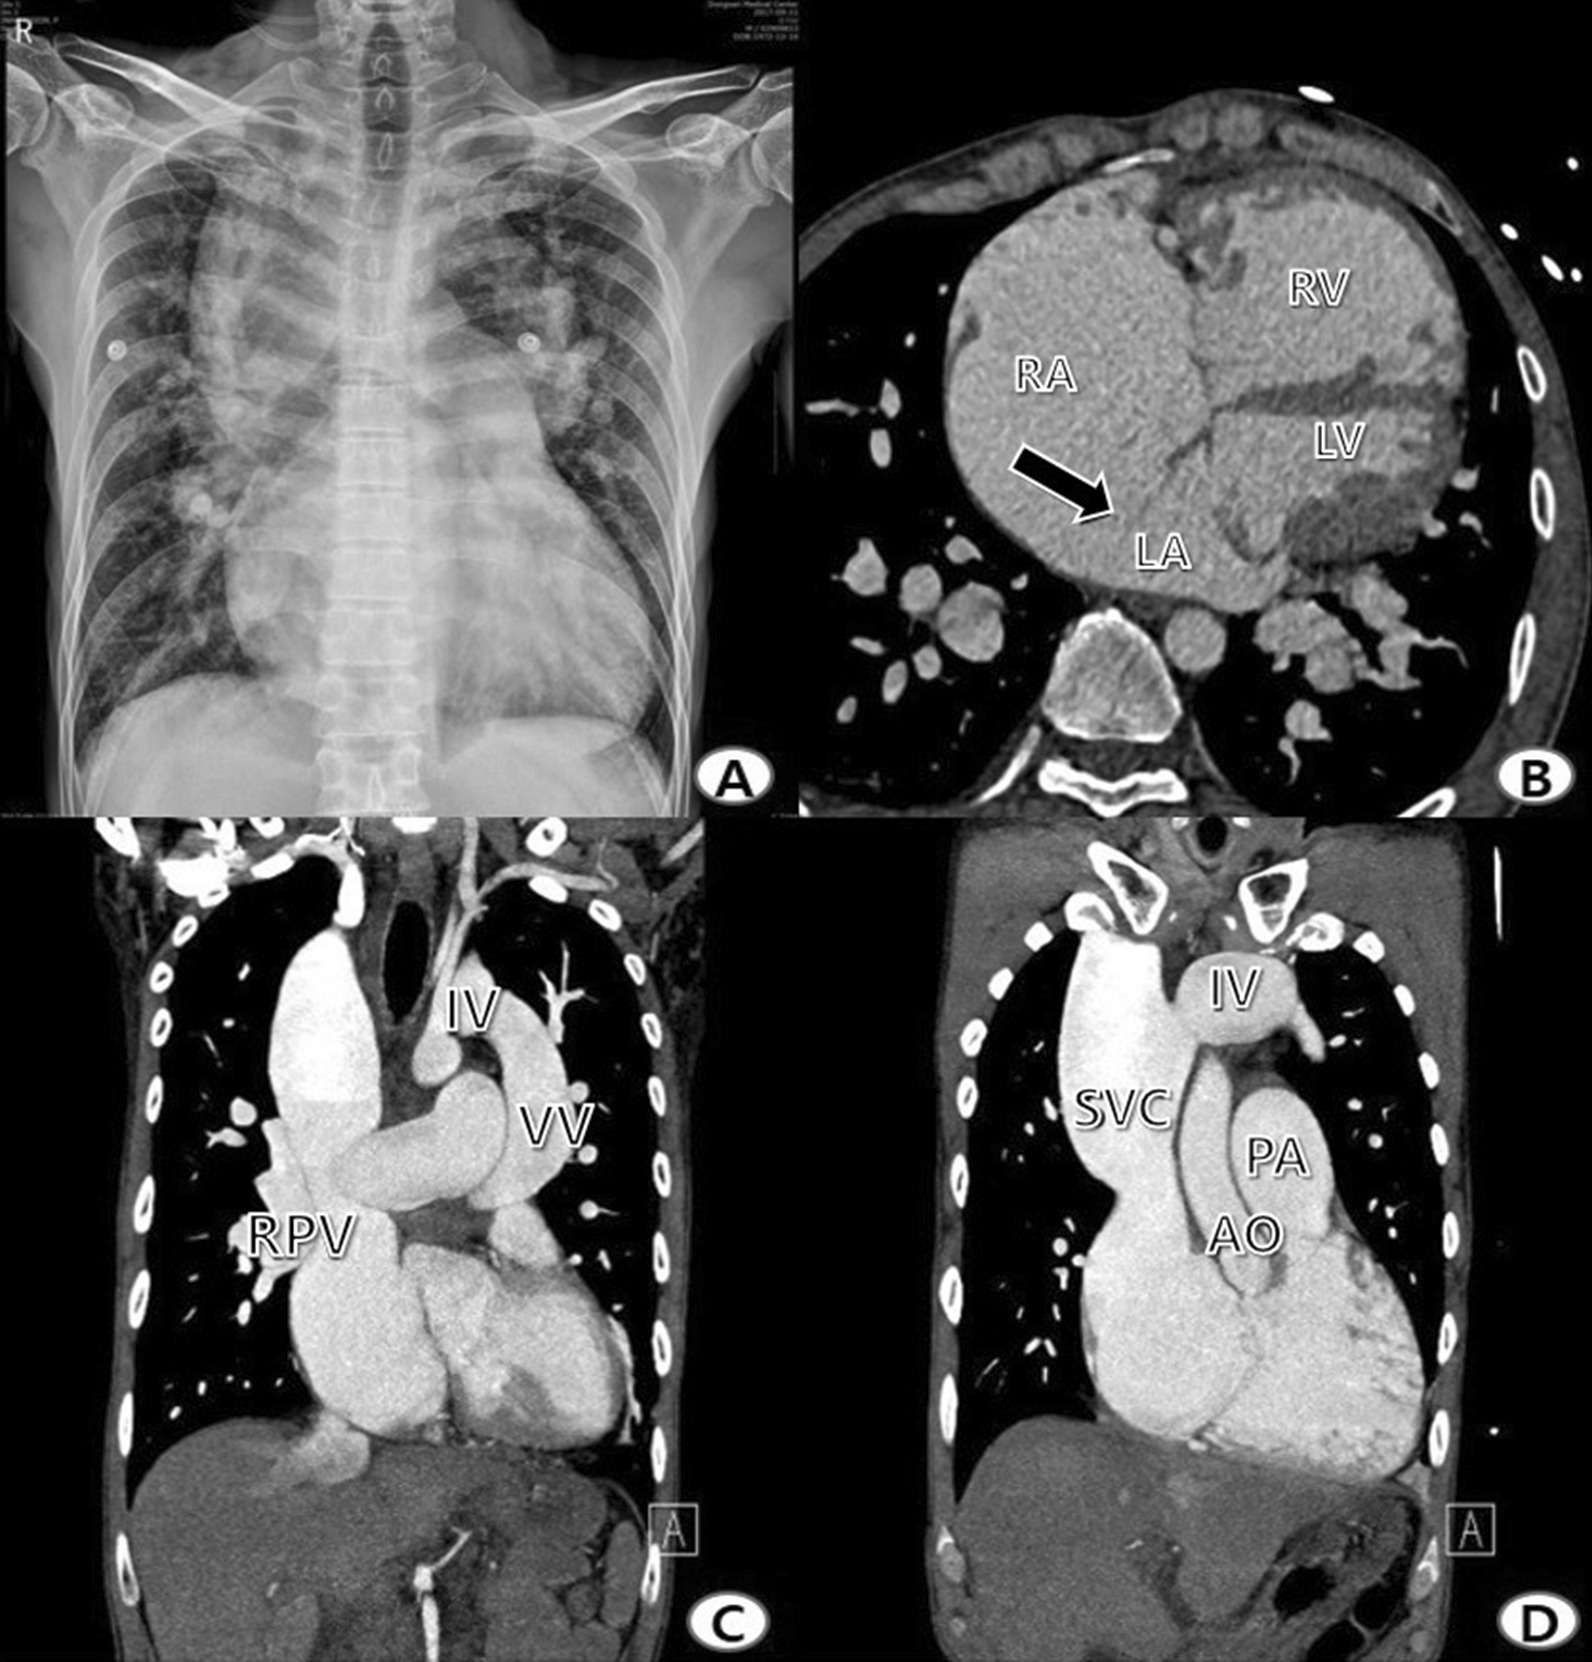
Preoperative chest X-ray and computed tomography (CT). A Preoperative chest X-ray. B Axial view on CT shows an enlargement of the RA and RV, secundum-type ASD (arrow), and left dislocation of the LA and LV. C, D Coronal view on CT shows that the IV is connected to the VV and drains to the SVC. RA, right atrium; RV, right ventricle; ASD, atrial septal defect; LA, left atrium; LV, left ventricle; IV, innominate vein; VV, vertical vein; RPV, right pulmonary vein; SVC, superior vena cava; PA, pulmonary artery; AO, aorta
